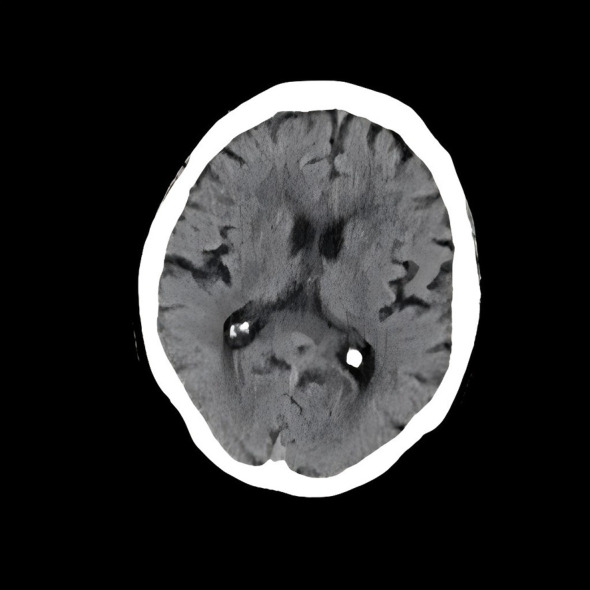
CT scan: Multiple cystic and patchy hypodense lesions in bilateral basal ganglia, corona radiata, and centrum semiovale. Reduced white matter density adjacent to bilateral lateral ventricles. Clear gray–white matter differentiation.
